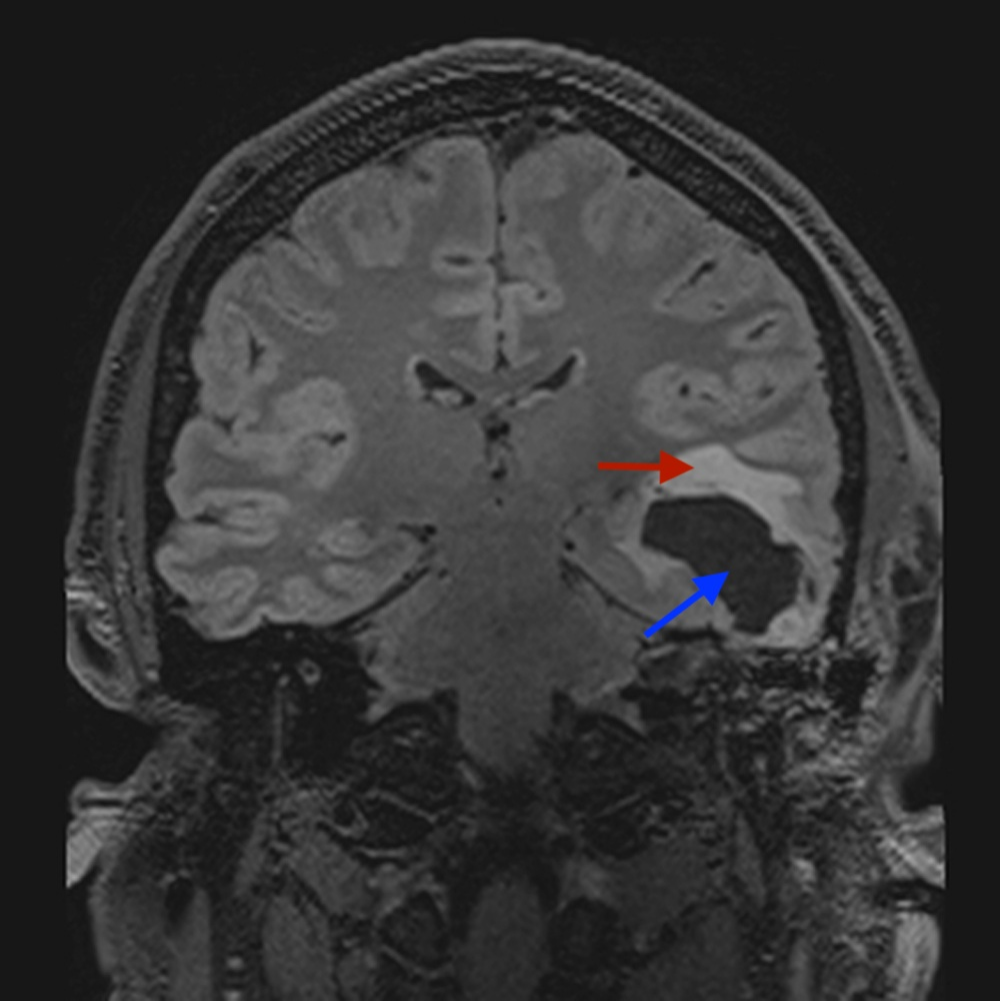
Coronal unenhanced T2 fat suppressed MRI of the brain: lucency in the temporal lobe (blue arrow) and associated surrounding oedema (red arrow). MRI - magnetic resonance imaging
